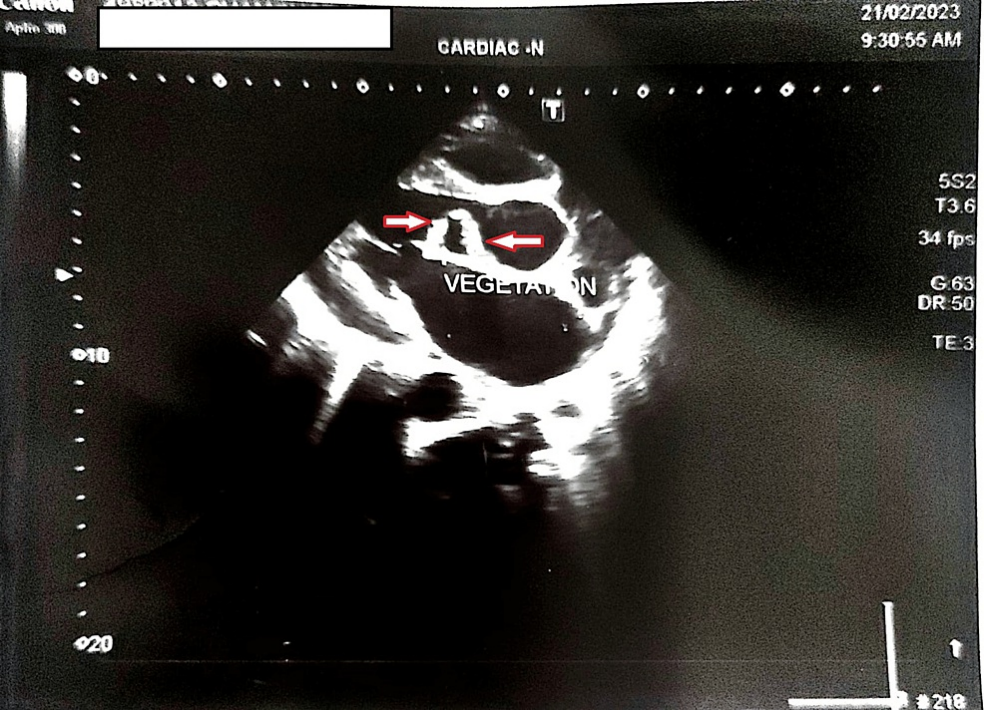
Echocardiogram showing multiple small aortic valve vegetations Red arrows indicate small multiple aortic vegetations
